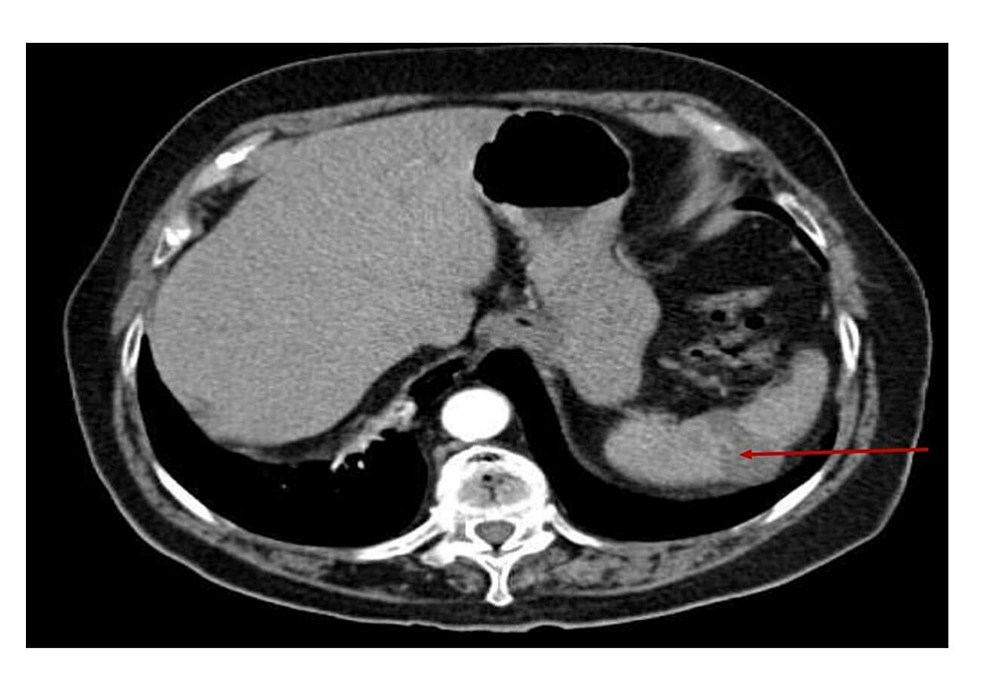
CT of abdomen and pelvis showing wedge-shaped infraction of spleen
